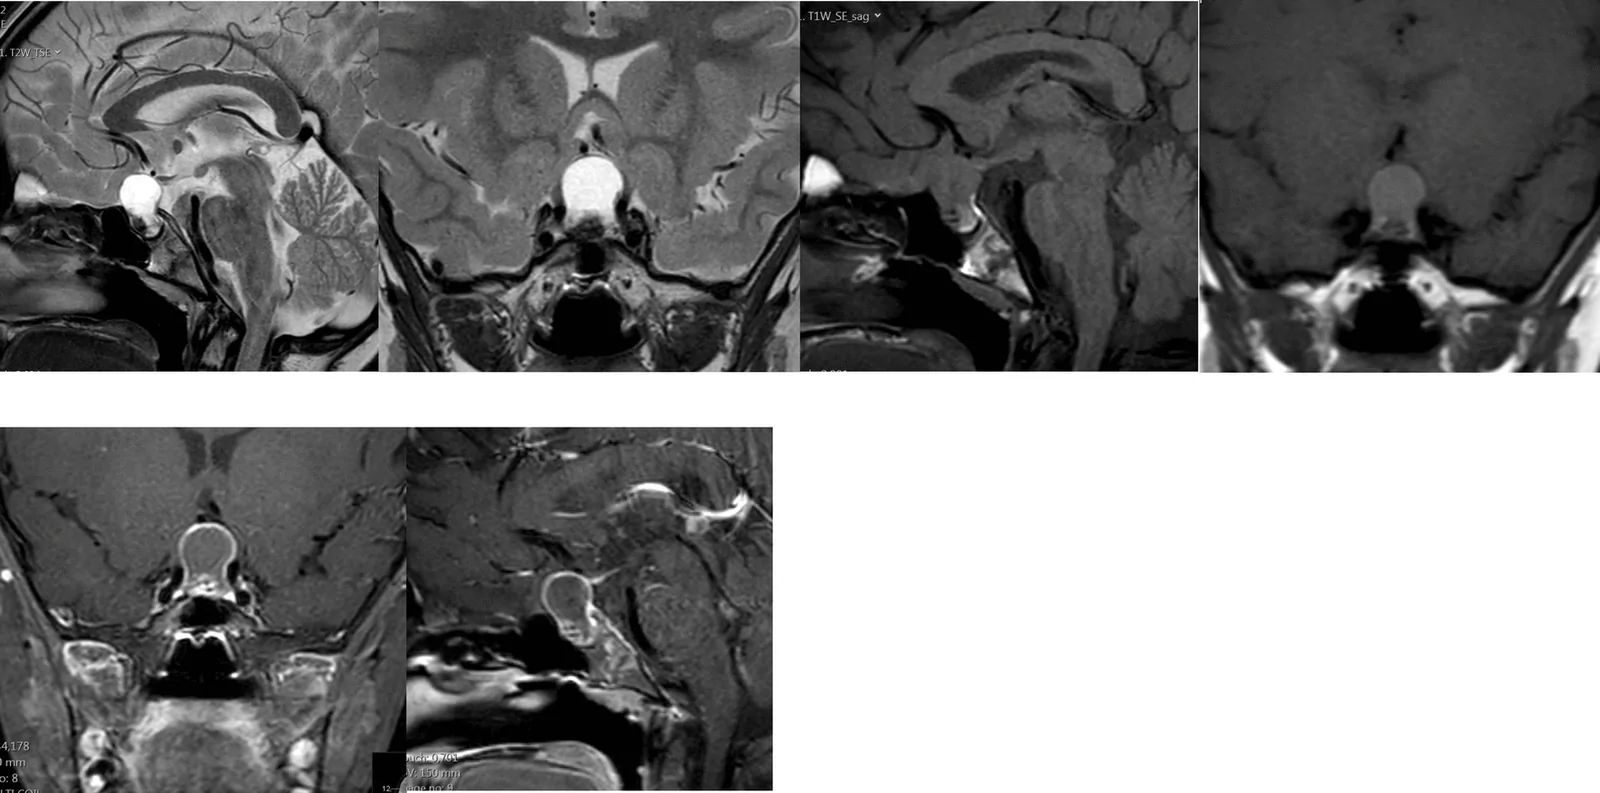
The case of a 10-year-old female with a surgically verified cystic craniopharygioma. Sagittal and coronal images of T2- and T1-weighted images (a-d) reveal a large and well-delineated sellar cystic lesion with a suprasellar extension. The lesion is hyperintense on a T2-weighted image and iso- to slightly hyperintense on the T1-weighted image. The lesion has a nodule in the cyst that displays low signal intensity on the sagittal and coronal T2-weighted images (a, b). The lesion shows wall enhancement on the sagittal and coronal contrast-enhanced T1-weighted images (e, f). Note that the nodule also shows contrast enhancement
